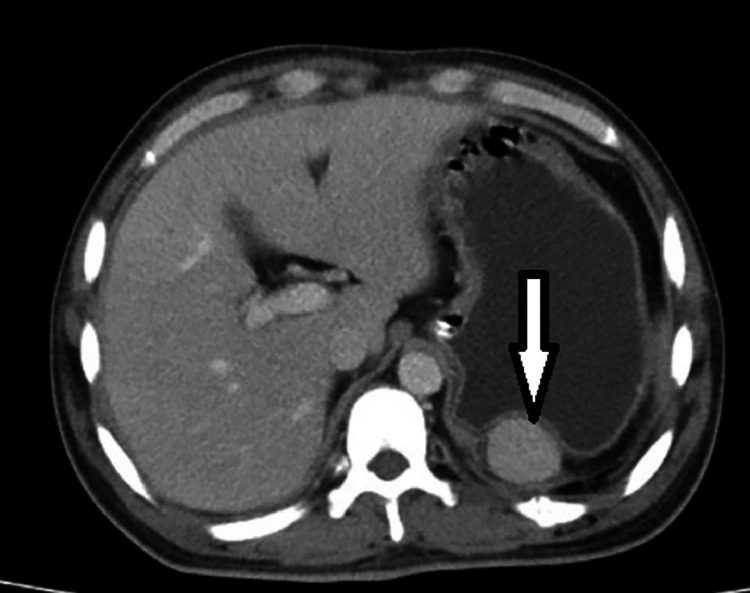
Axial contrast enhanced CT image showing absence of spleen and splenic implant in left hypochondrium.
